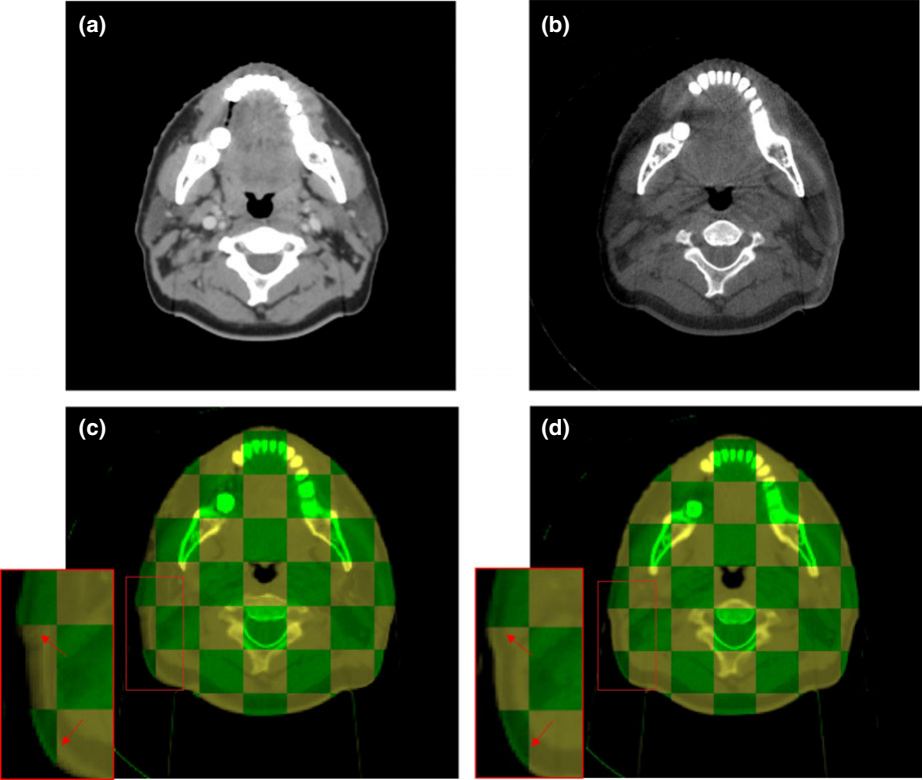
One example of the comparison between the GFFD algorithm and the Demons algorithm; (a) is the planning CT image; (b) is the corresponding CBCT image; (c) is the checkerboard comparison between the fixed image (CBCT) and the registered image (mCBCT) after Demons registration; (d) is the checkerboard comparison between the fixed image (CBCT) and the registered image (mCBCT) after GFFD registration. In (c) and (d), the checkerboard in green indicates the fixed image, and the checkerboard in yellow indicates the registered image.
